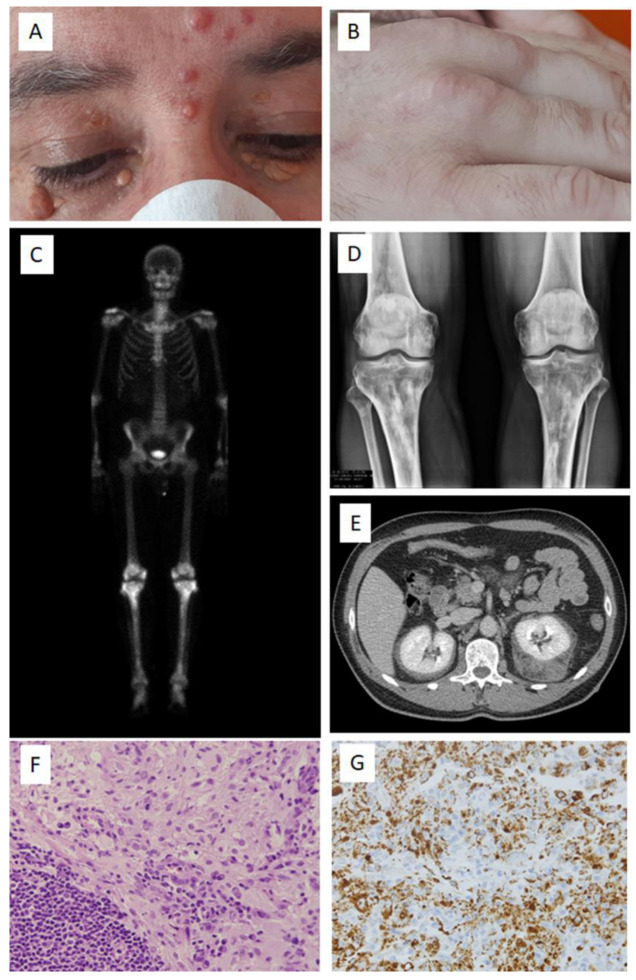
(A) Yellowish xanthelasma at the eyelids, as well as several reddish papulo-nodular lesions. (B) Tendinous xanthoma over the metacarpal joints. (C) Bone scintigraphy showing increased uptake at several metaphyseal regions, more intense in the distal femora and proximal tibia. (D) Plain X-ray showing mixed lesions in the femora and tibia. (E) Contrast-enhanced CT scan showing a peri-renal infiltration, more marked around the left kidney. (F) Biopsy of a nodular skin lesion showing diffuse interstitial accumulation of histiocytes with xanthomatous features and a nodular lymphoplasmacytic infiltrate (×400). (G) CD68-positive histiocytes (×200).
